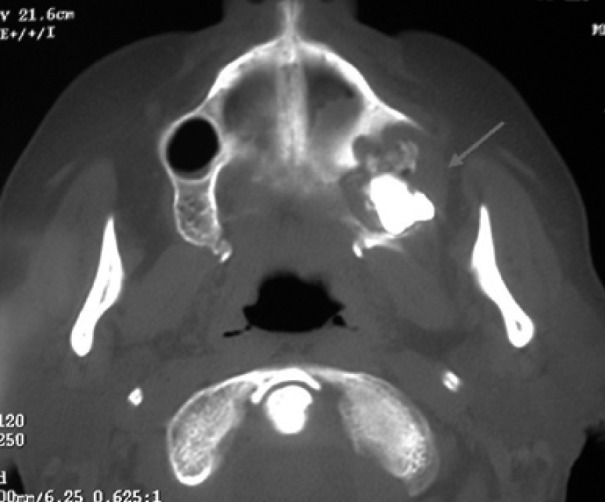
Axial CT image showing large sequestrum around third molar included (red arrow).
